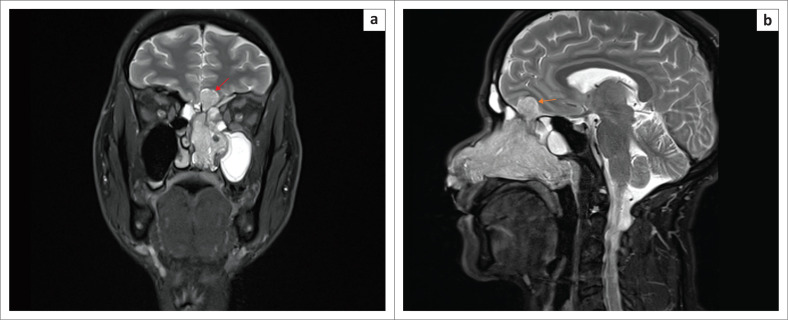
(a) Coronal T2 turbo spin echo (TSE) with fat saturation (FS) MRI. There is an expansile T2 solid hyperintense polypoid mass extending intracranially into the anterior cranial fossa with mild mass effect on the inferior left frontal lobe (red arrow). The maxillary sinus is obstructed with a resultant effusion. (b) Sagittal T2 turbo spin echo (TSE) with fat saturation (FS) MRI image shows the antero-posterior extent of the mass, the extradural intracranial component (orange arrow) and obstructed frontal and sphenoid sinuses.
